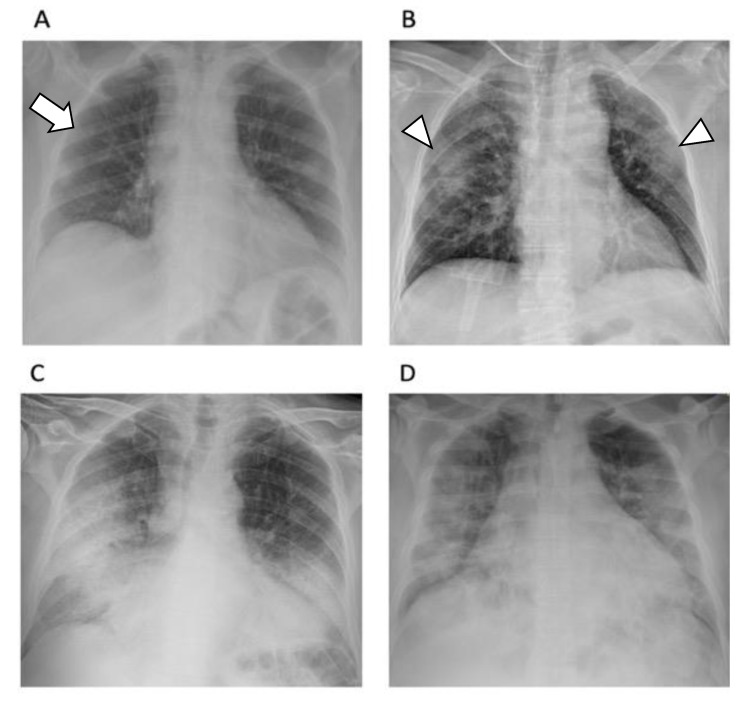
Chest X-ray of patients affected by SARS-CoV-2 according to the extent of lung abnormalities involvement. (A). Lung involvement ≤ 25% (arrow). (B). Lung involvement 25–50% (arrowheads). (C). Lung involvement 50–75%. (D). Lung involvement ≥ 75%.
